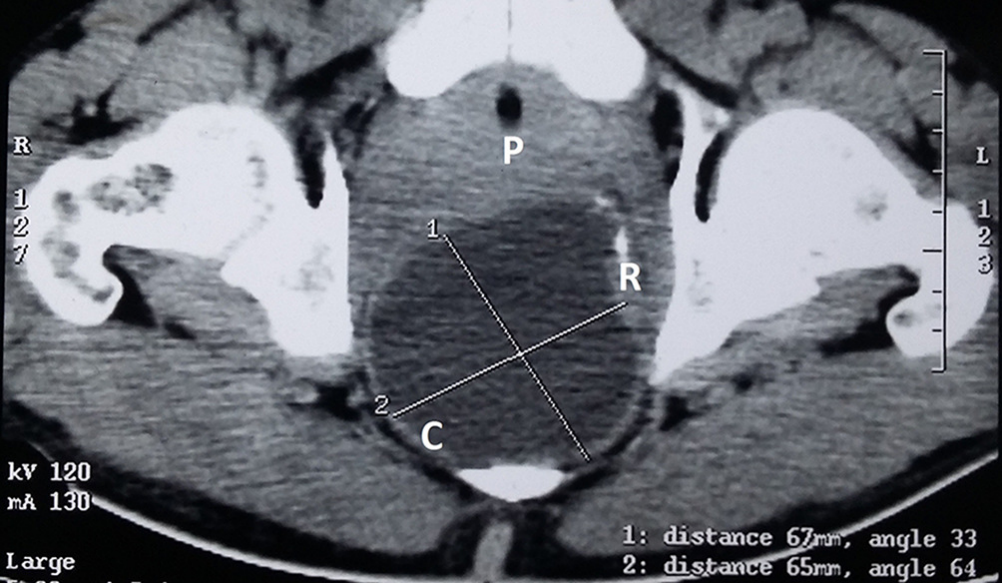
CT CE axial: A well-defined thin-walled smooth cystic SOL (C) compressing the anteriorly displaced rectum (R). UB, urinary bladder.
